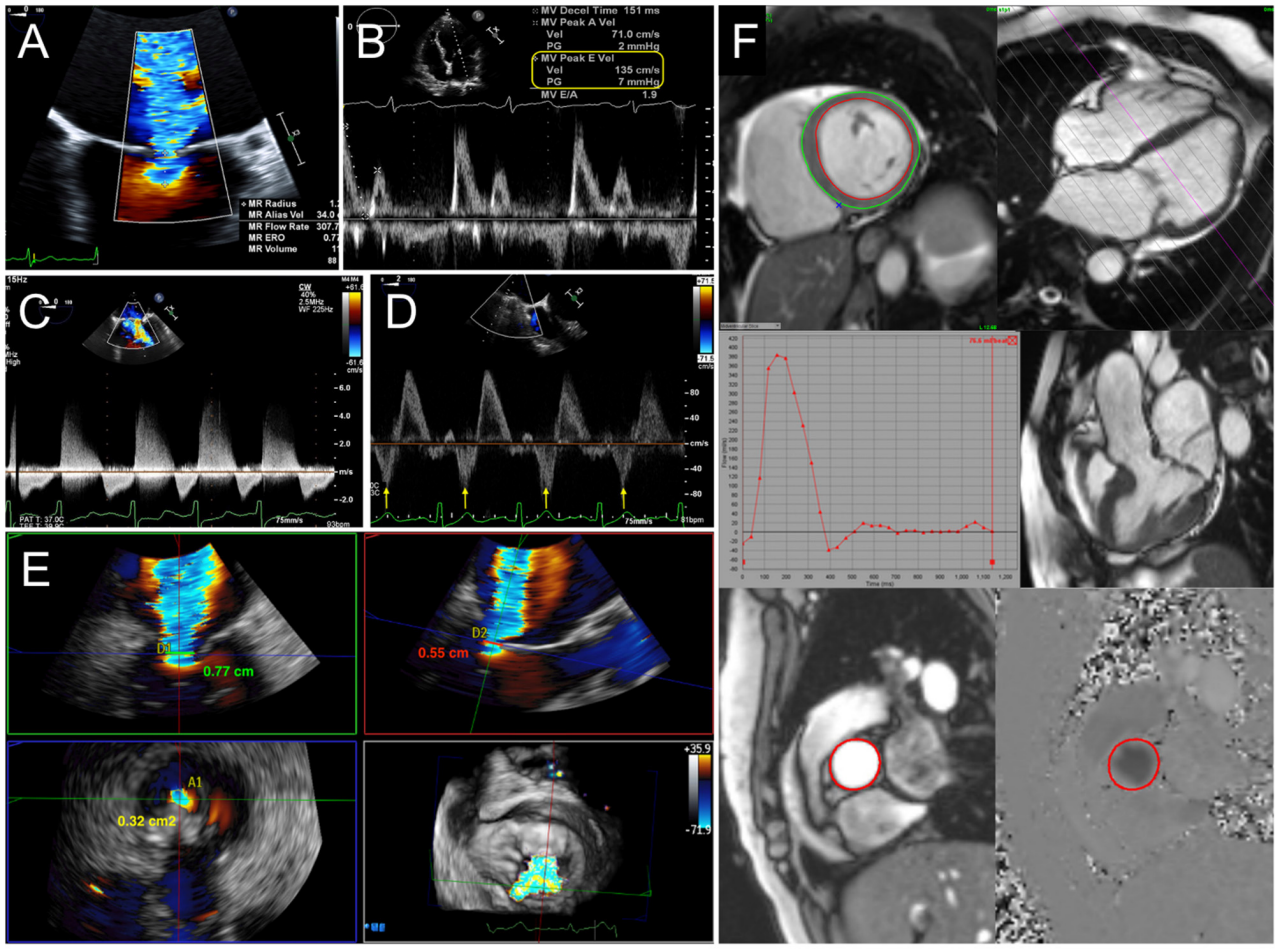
Quantification of mitral regurgitation (MR). (A) Calculation of effective regurgitant orifice area (EROA) and regurgitant volume (RVol) by proximal isovelocity surface area (PISA) method. Indicators for severe MR include elevated early diastolic mitral inflow (E wave) velocity (B), dense continuous-wave Doppler signal (C), and systolic reversal of pulmonary vein flow (D). (E) 3D vena contracta area reconciles discrepant linear vena contracta width measurements in an elliptical regurgitant orifice. (F) With cardiac MRI, mitral RVol is calculated as the difference between total left ventricular stroke volume and the aortic flow (forward stroke volume) by phase-contrast imaging.
